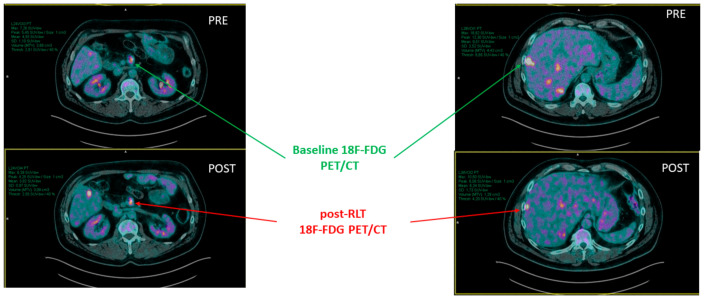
The figure shows the direct comparison made between baseline and post-RLT FDG PET/CT scans, in terms of SUVmax variation (ΔSUVmax) for target 2 and target 3. In this patient, target 1 was not evaluated since the patient had received surgery on the primary lesion.
